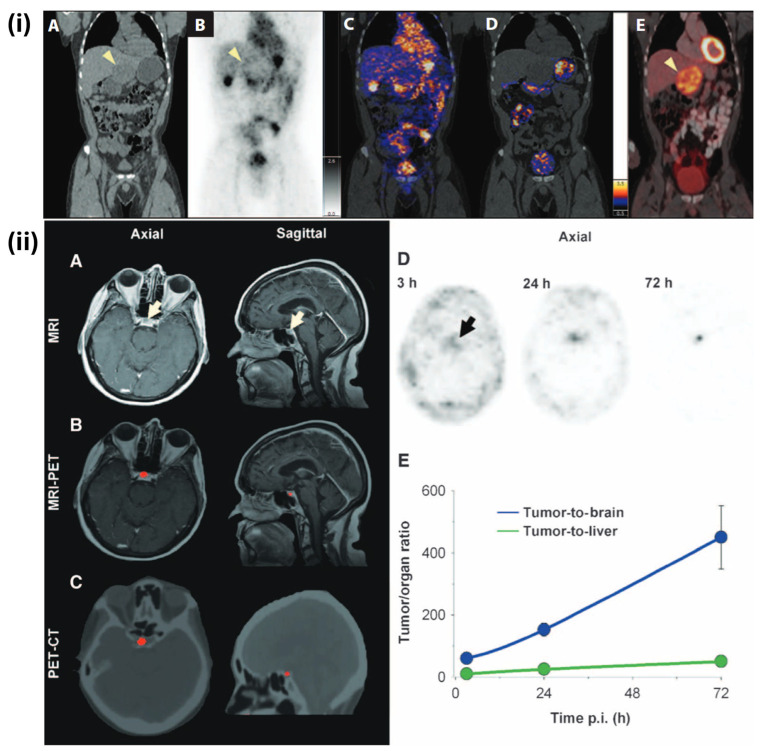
Clinical PET-based tracking and multimodal imaging of ^124^I-cRGDY–PEG–C dots. (i) Whole-body PET/CT in a patient with a hepatic metastasis: (A) Reformatted coronal CT demonstrates a hypodense left hepatic lobe metastasis (arrowhead); (B) Coronal PET image at 4 h post-injection demonstrates particle activity along the peripheral aspect of the tumor (arrowhead), in addition to the bladder, gastrointestinal tract, gallbladder, and heart; (C) Co-registered PET/CT at 4 h post-injection localizes activity to the tumor margin; (D) Co-registered PET/CT at 24 h post-injection localizes activity to the tumor margin; (E) Corresponding 18F-FDG PET/CT image showing the hepatic metastasis (arrowhead). Color and gray scales reflect SUV values. (ii) Multimodal imaging of a pituitary lesion: (A) Multiplanar contrast-enhanced MR axial and sagittal images at 72 h post-injection demonstrate a subcentimeter cystic focus (arrows) within the right aspect of the anterior pituitary gland; (B) Co-registered axial and sagittal MRI–PET images reveal increased focal activity localized to the lesion site; (C) Axial and sagittal PET/CT images localize activity to the right aspect of the sella; (D) Axial PET images of the brain at 3, 24, and 72 h post-injection demonstrate progressive accumulation of activity within the sellar region; (E) Tumor-to-brain and tumor-to-liver activity ratios as a function of post-injection (p.i.) time. Adapted with permission from ref. [16]. Copyright 2014, American Association for the Advancement of Science.
